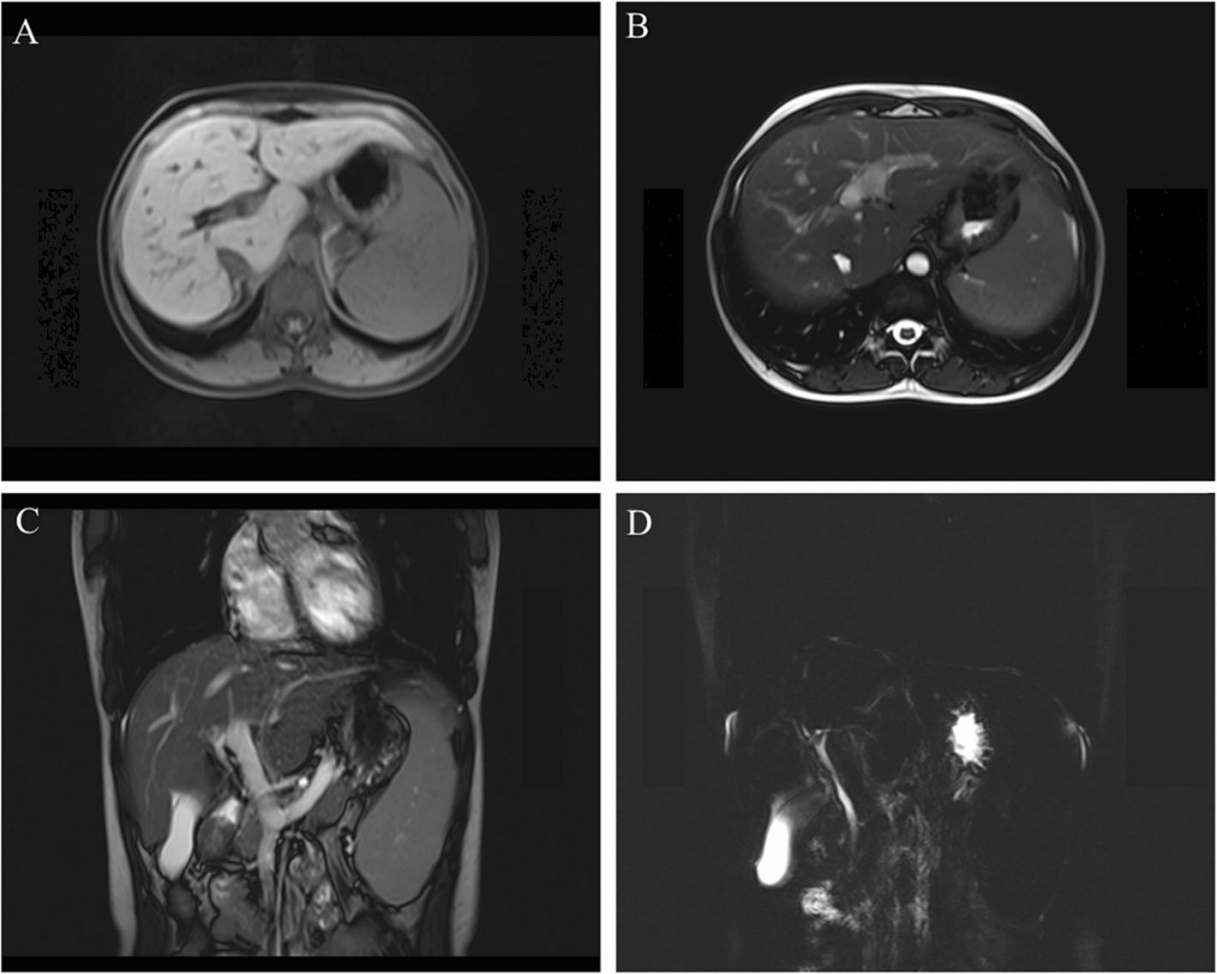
Liver magnetic resonance image. The abdominal magnetic resonance image shows liver cirrhosis, portal hypertension, splenomegaly, and cholestasis. No obstruction of the intrahepatic and extrahepatic bile ducts was found after further magnetic resonance cholangiopancreatographic examination
